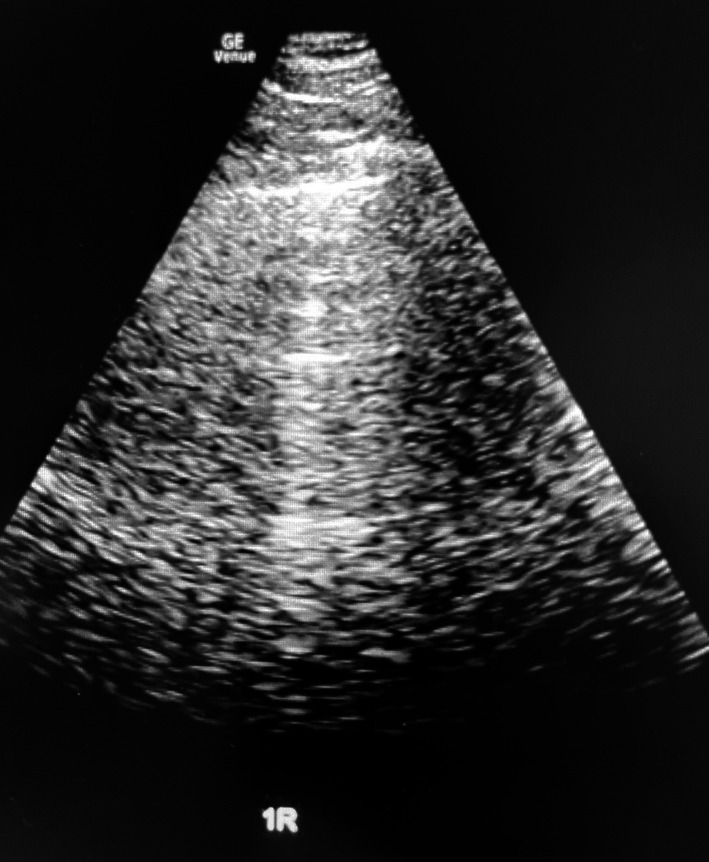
Lung ultrasound image from the right anterior chest showing horizontal A‐lines with absence of B‐lines or pleural effusion, consistent with the absence of interstitial pulmonary edema.
